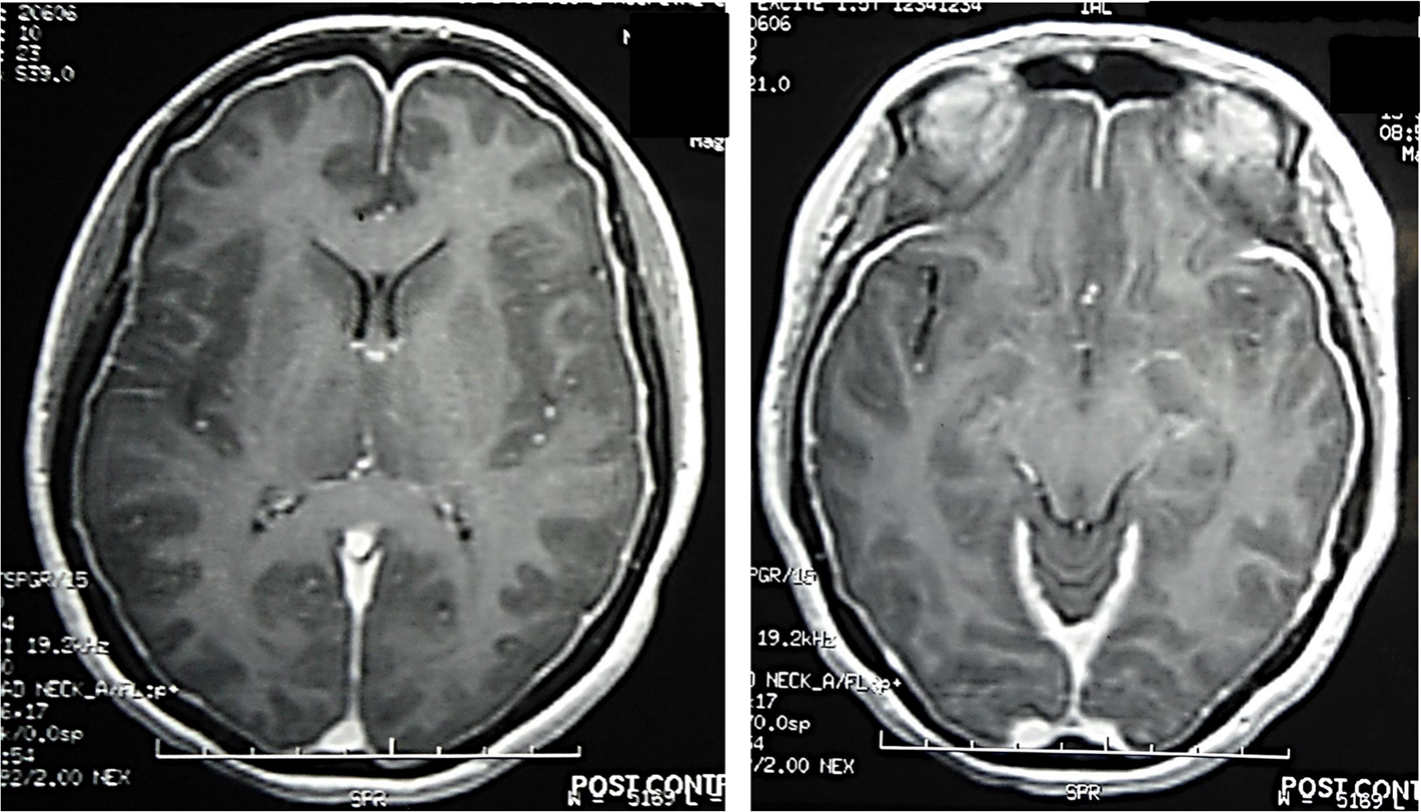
Gadolinium-enhanced cranial magnetic resonance imaging showing generalized, uniform pachymeningeal enhancement.
